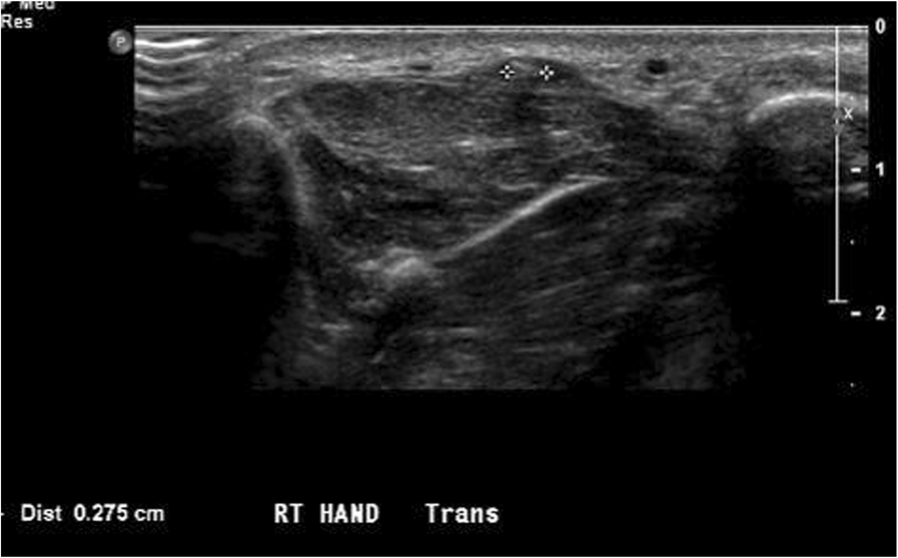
High frequency linear ultrasound image. Ultrasound detection of a 2.7 mm wooden splinter in the first webspace distance measurement in between the 2 tracking cross marks. This demonstrates the resolution of current ultrasound probes which would have allowed for easy detection of the nearly 3 cm or 1 inch long splinter shown in Figure
